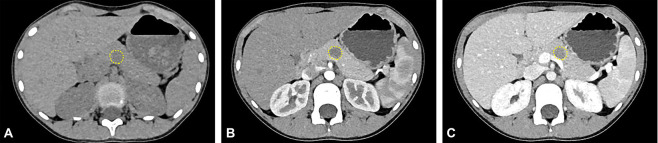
(Case 3): Non-contrast CT demonstrates a slightly hypodense lesion at the junction of the pancreatic body and neck (a, yellow circle), which shows progressive enhancement during the arterial phase (b) and venous phase (c) on contrast-enhanced CT (yellow circle).
